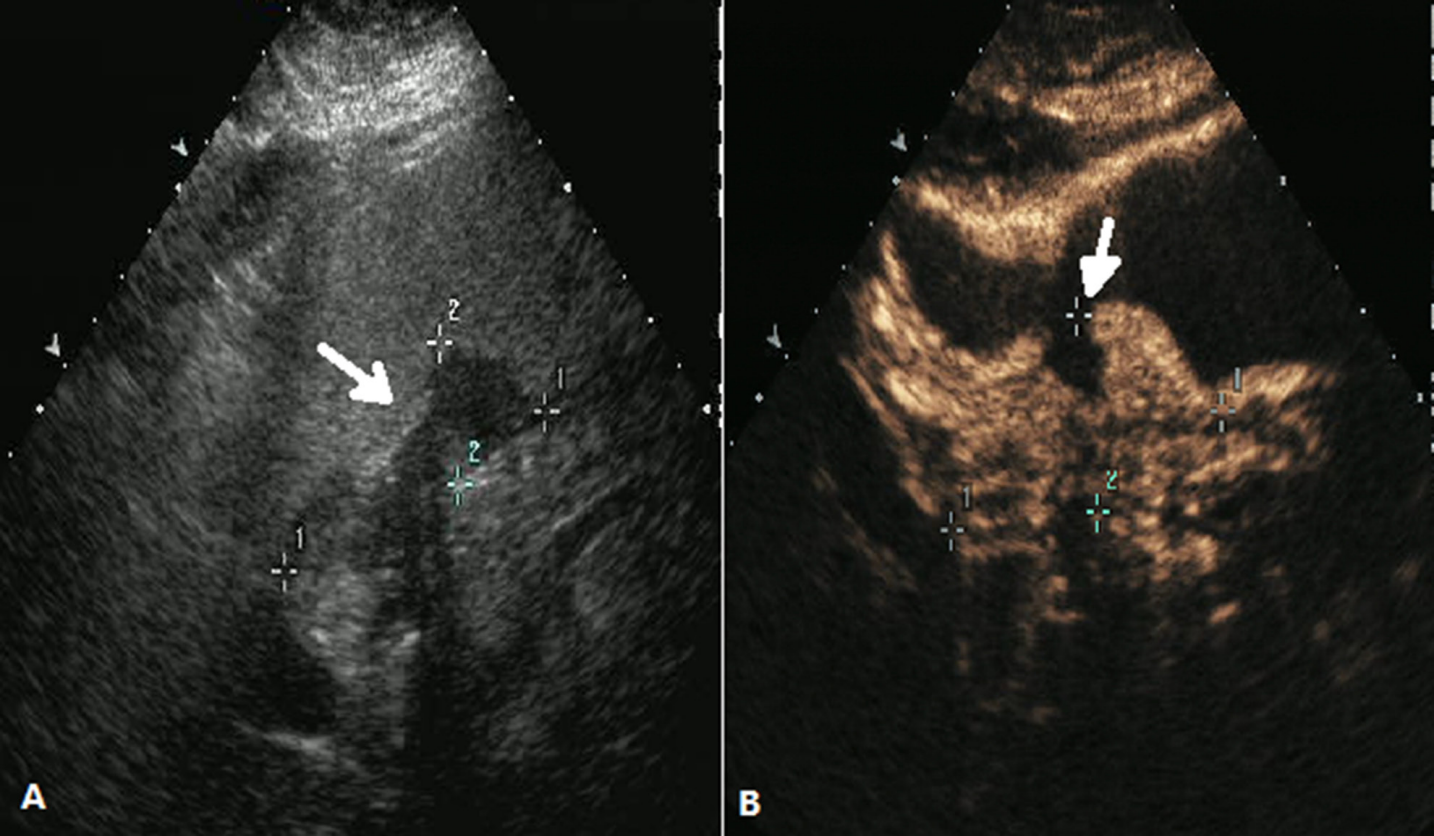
DCEUS images of a case classified as Borrmann type III (A) Ultrasonography showed the ulcerating lesion with invasion of the gastric wall (arrow) after the stamoch was filled with UOCA, (B) showed the lesion(arrow) was enhanced after administration of Sonovue.
